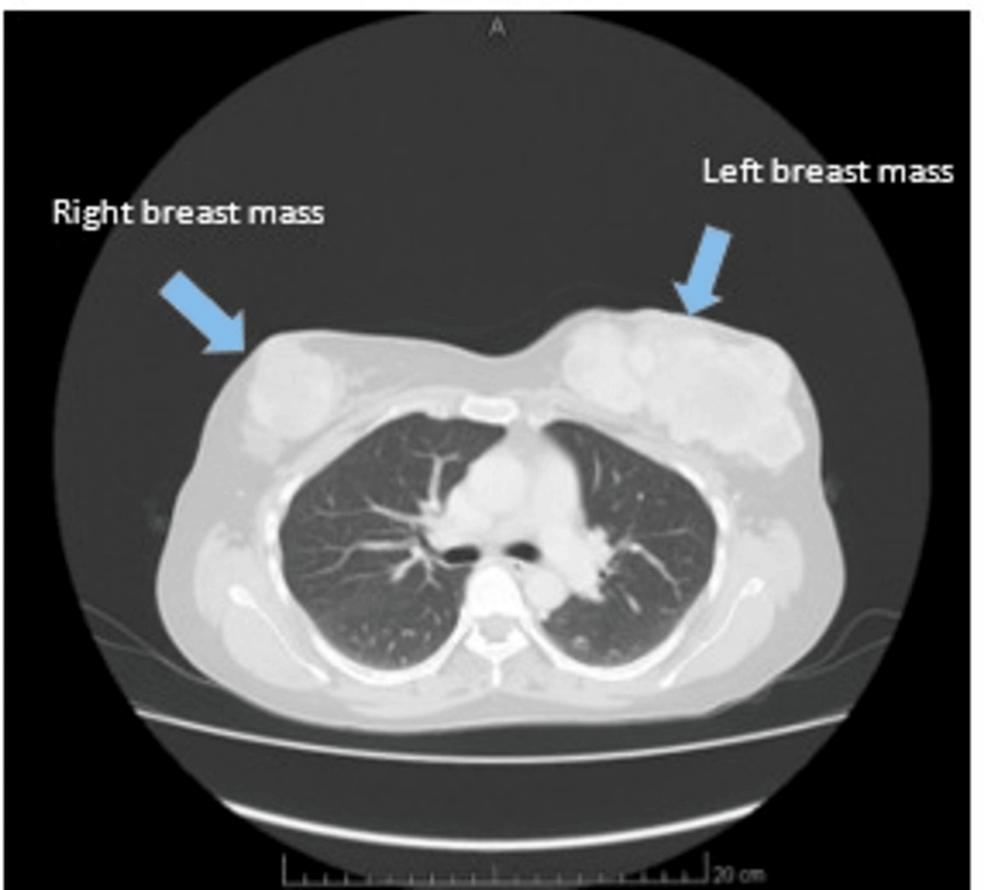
CT imaging (taken October 2019) demonstrated multiple well-defined soft tissue masses with areas of necrosis, with the largest lesion measuring 7.3 x 5.4 cm in the left breast.
